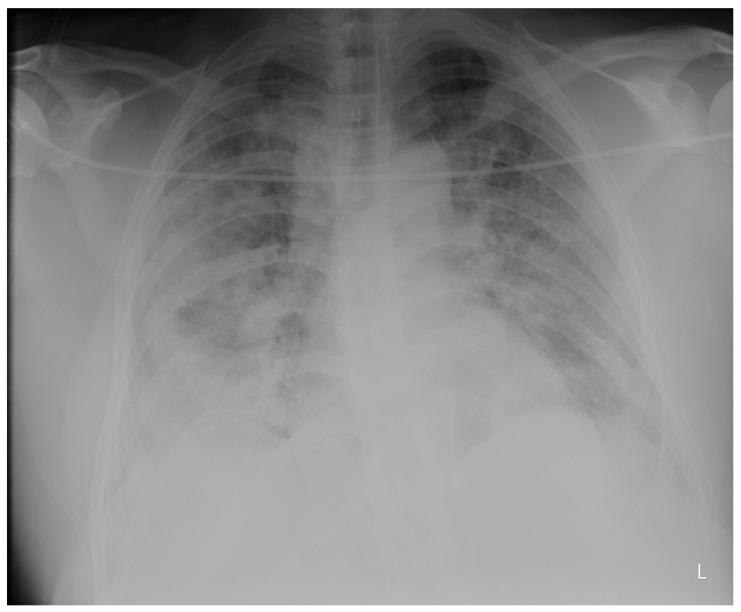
Chest X-ray upon admittance: patient 5. Bilateral inhomogenous infiltrates typical for COVID-19 pneumonia (the “L” orientation marker indicates the left side).
